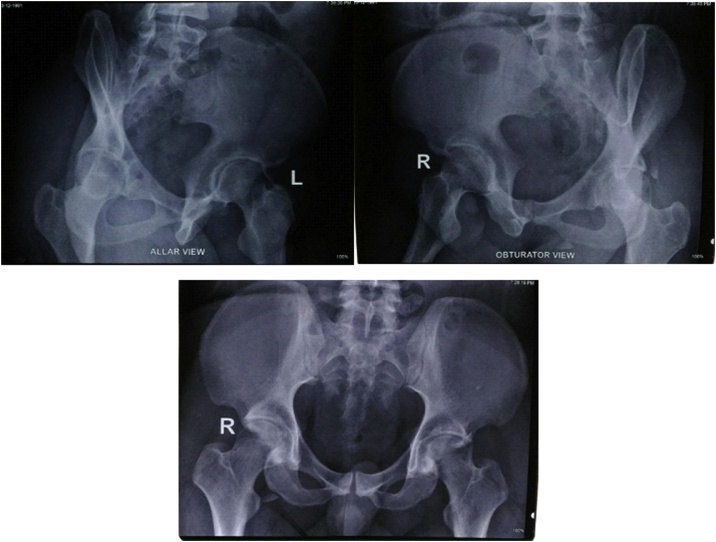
X-Ray After Closed Reduction; A) Allar View; B) Obturator View; C) Anteroposterior View.
